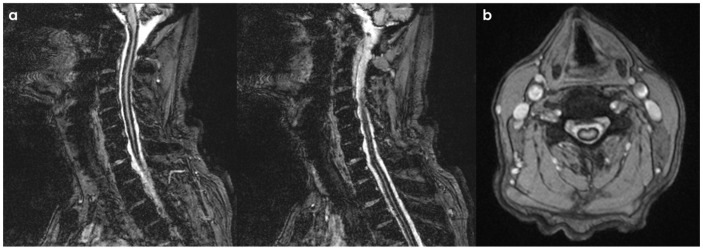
T2W sagittal (a) and axial (b) MRI at the cervical level with extensive circumferential hypointense lining of brainstem and spinal cord, suggestive for siderosis.
